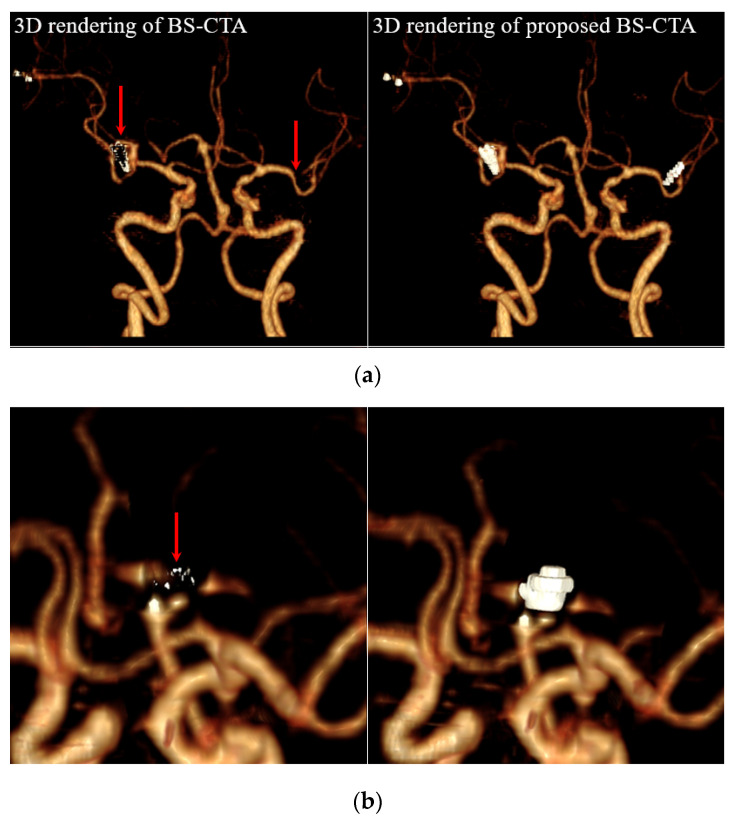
Results of 3D rendering based on BS-CTA and proposed BS-CTA from Figure 7. (a) Insertion of the two clips and (b) the coil components.
